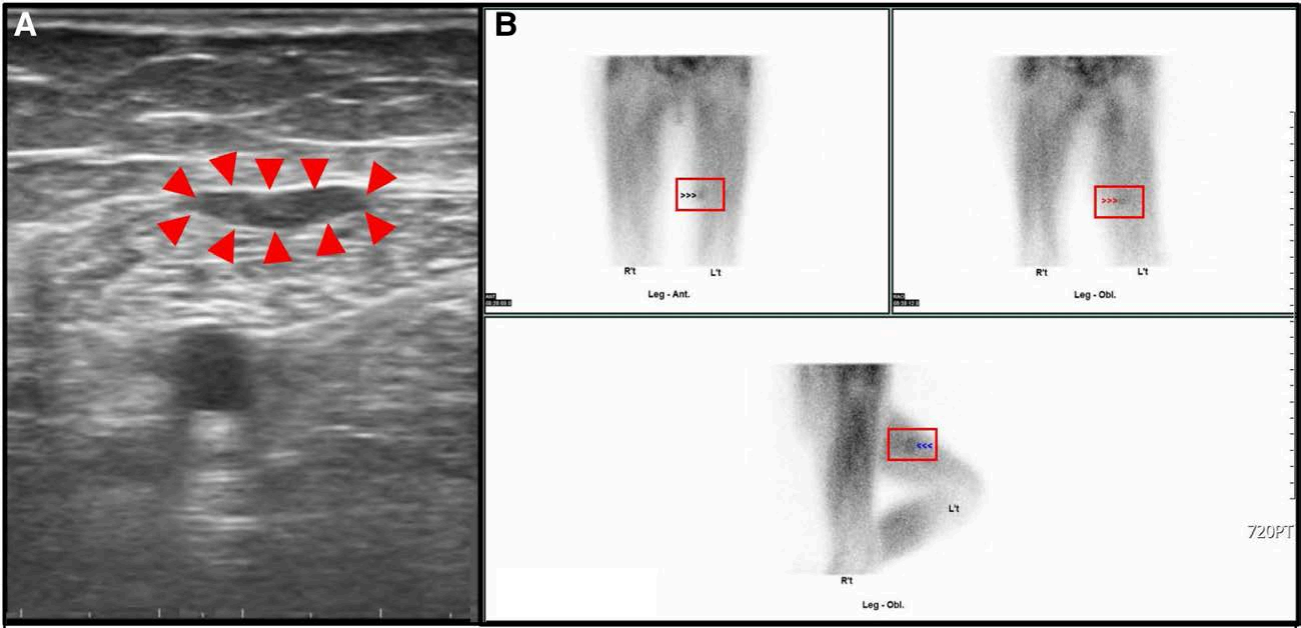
A. 2 hypoechoic lesions (1.75 cm × 0.57 cm, arrow head) respectively were noted on the left medial thigh in intraoperative sonography before second operation. Figure 3B. The dual phase Tc-99m MIBI parathyroid scintigraphy showed abnormal uptake on left thigh before second operation. (square frame).
